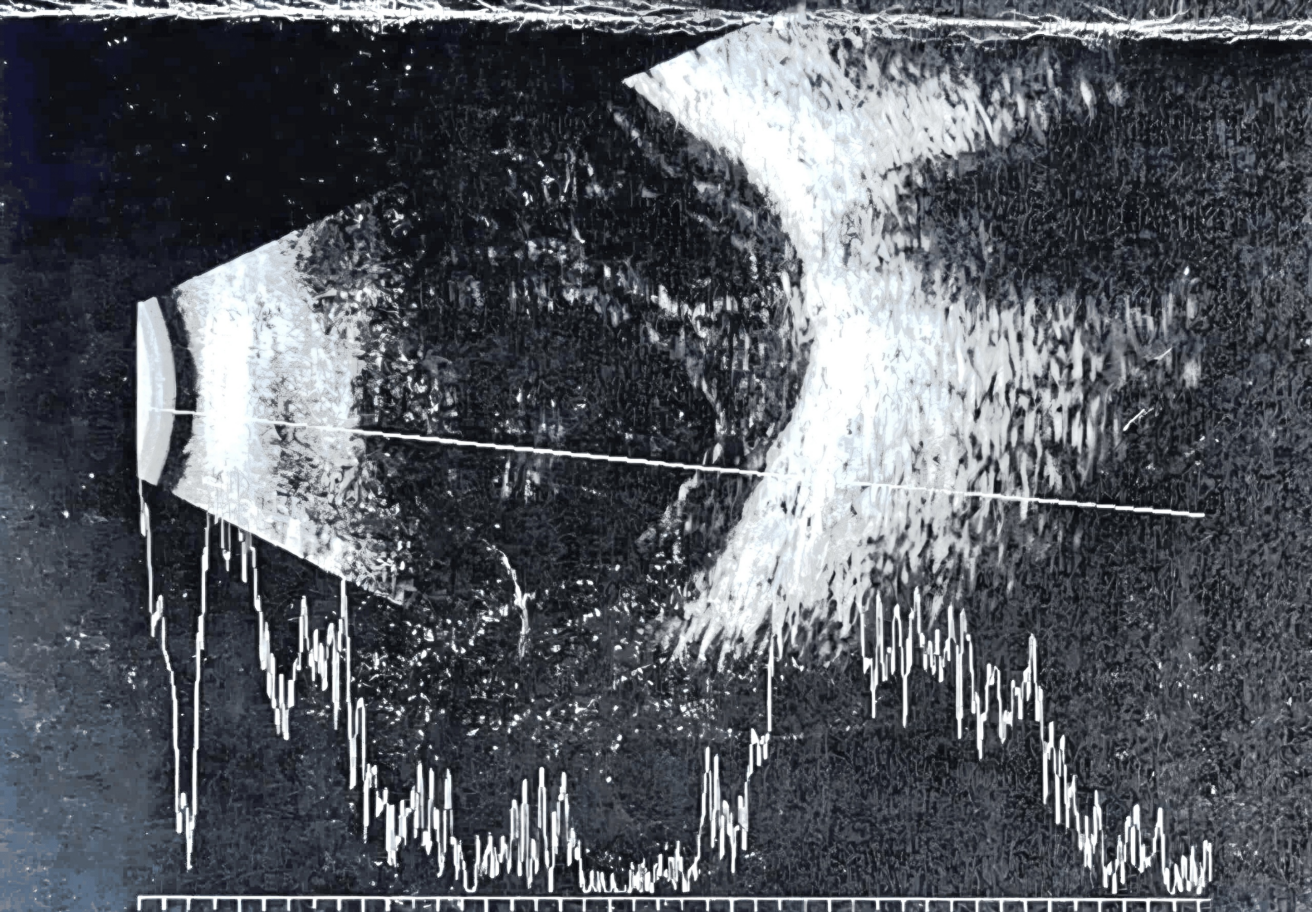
B-scan of the right eye showed vitreous opacities with loculations; retina flat B-scan: brightness scan ultrasonography
